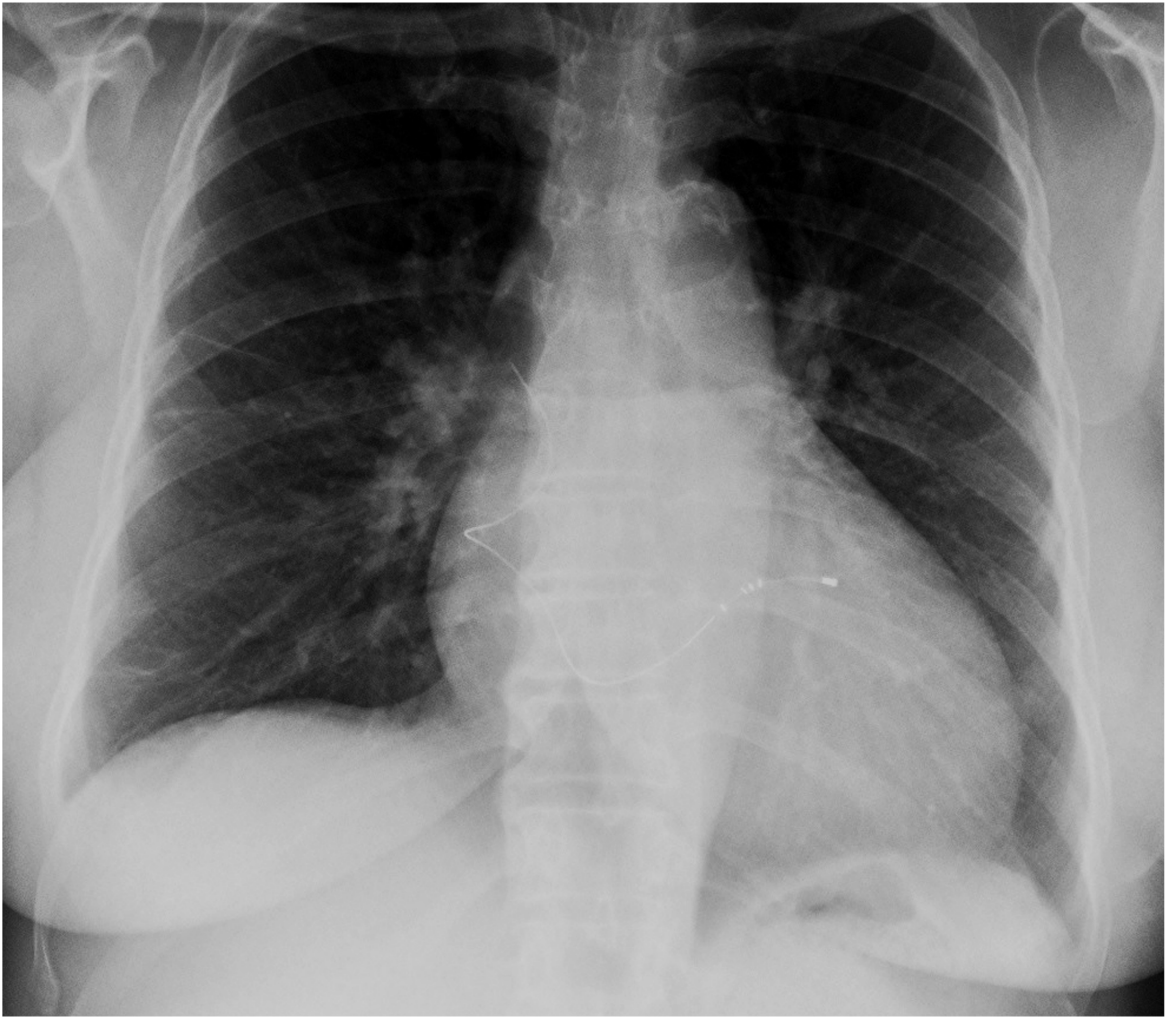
Chest-X-Ray with abandoned LV Lead fragment after extraction failure.
